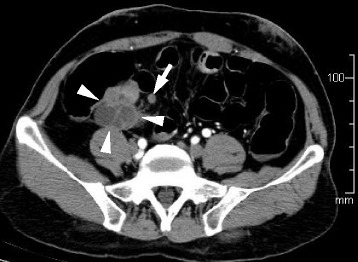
CT findings. Abdominal CT showed polycystic tumor in the ileocecal area (*arrowhead*) and a swollen lymph node (*arrow*)
